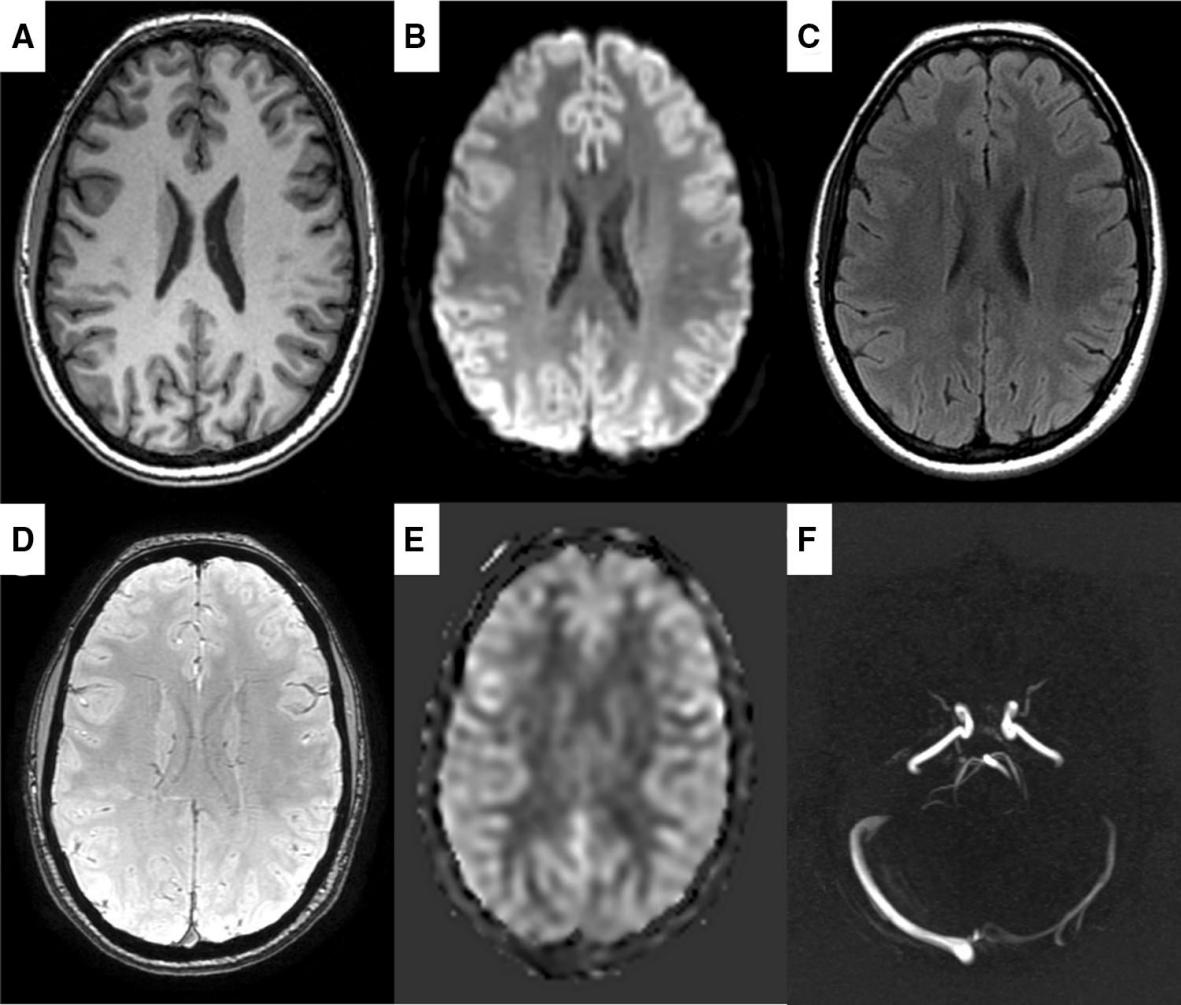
Example images from all sequences in the MRI protocol. a T_1_-weighted, b diffusion-weighted, c FLAIR, d T_2_*-weighted, e pcASL, f phase contrast
